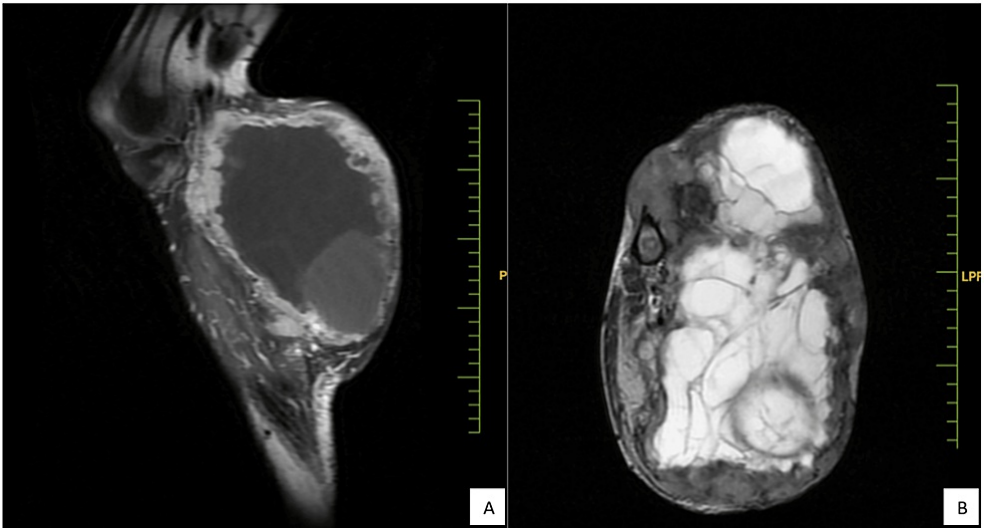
MRI left leg (A) sagittal view and (B) axial view MRI: magnetic resonance imaging
